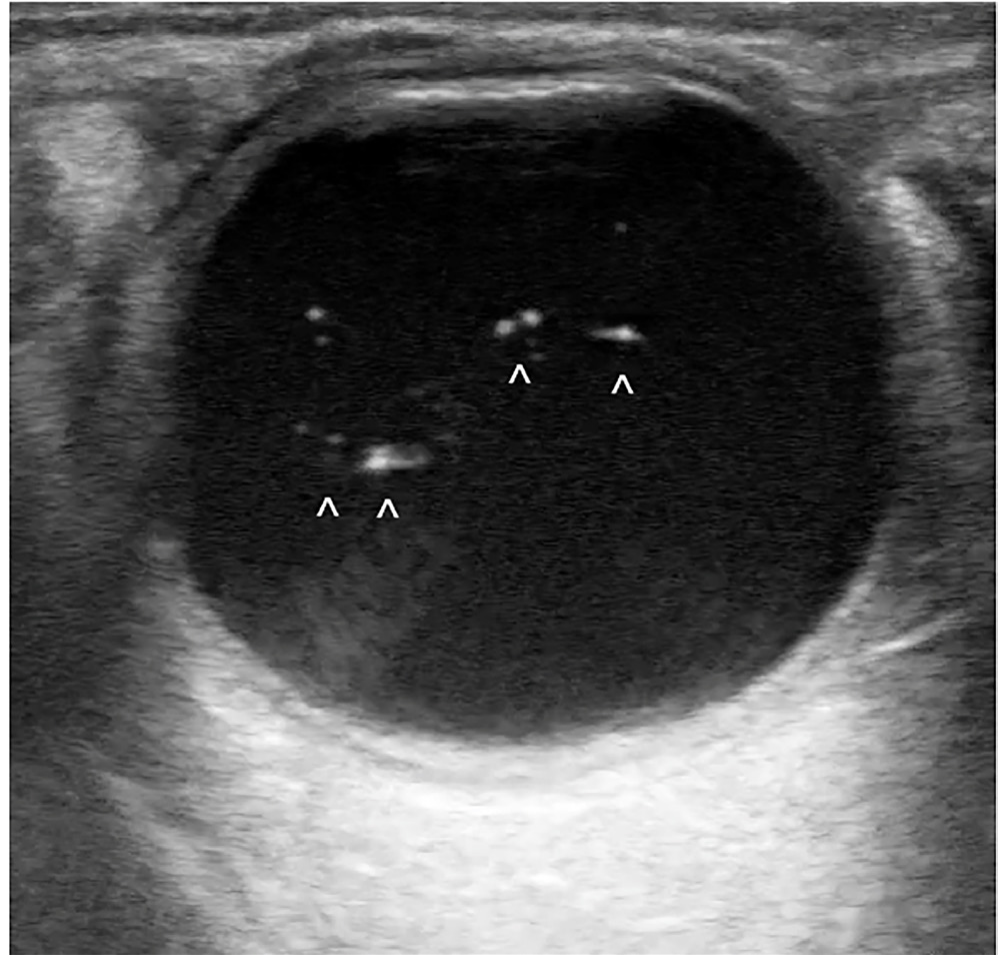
B-mode sonogram demonstrating several discrete, free-floating, echogenic particles (^) consistent with asteroid hyalosis.
